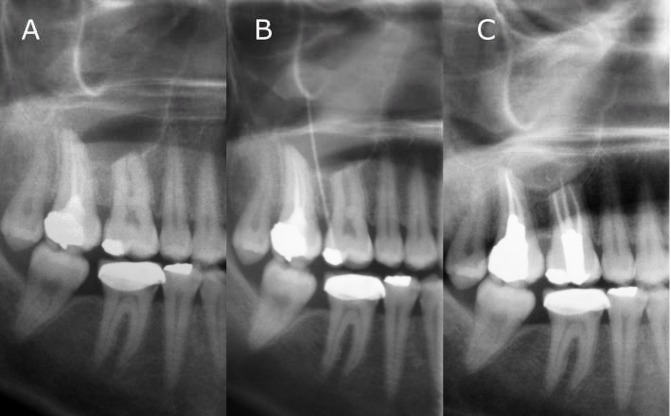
Panoramic radiography demonstrating: A) First and second right maxillary molars probably responsible for the cyst-like lesion appearing at their apices, B) Gutta-percha used for tracing the course of sinus tract showing occluso-apical dimension of the lesion which was not detected in periapical view, and C) Complete healing of the lesion 9 months after root canal therapy and permanent restoration
